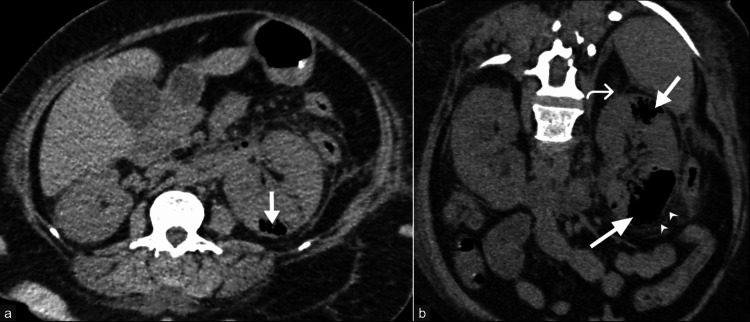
Class 3A emphysematous pyelonephritis with perinephric extension. A 50-year-old woman was diagnosed as Class 3A emphysematous pyelonephritis (as per the Huang and Tseng classification [9]). (a) Non-contrast axial CT KUB image shows multiple low-attenuation air foci (arrows) within the parenchyma and perinephric space of the left kidney, along with the thickening of renal fascia. (b) Coronal reformatted image shows large air pockets in the upper and lower poles of the left kidney with parenchymal destruction and extensive perinephric fat stranding and fluid (arrowheads). These images are from the hospital and have not been published elsewhere. CT, computed tomography; KUB, kidney, ureter, and bladder
